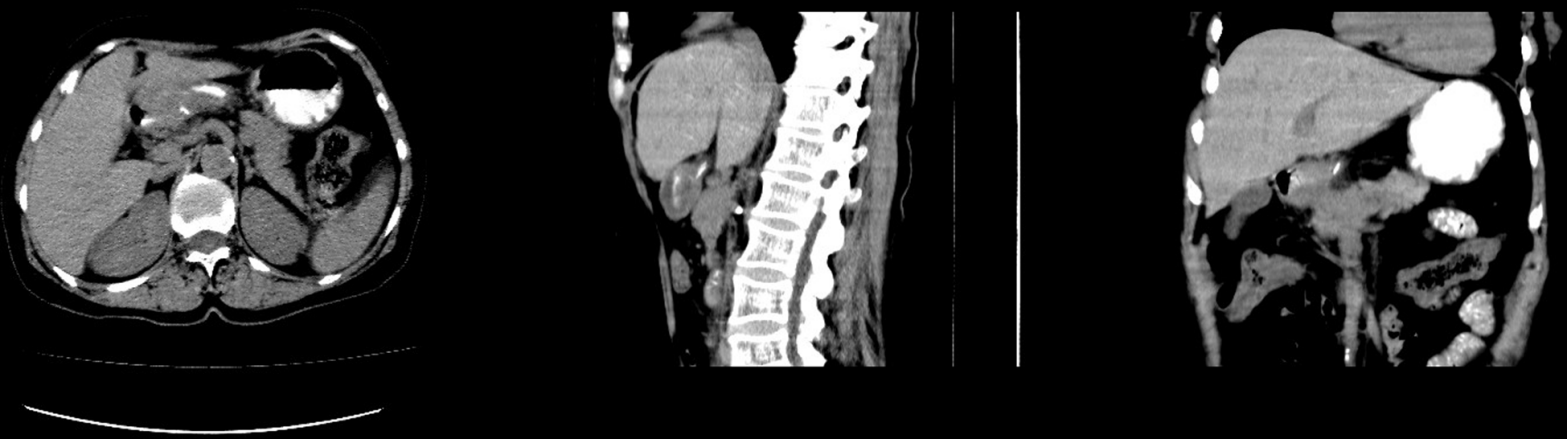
CT scan showed a streaky high-density shadow on the posterior wall of the gastric antrum extending outside the wall, with a length of about 3 cm
